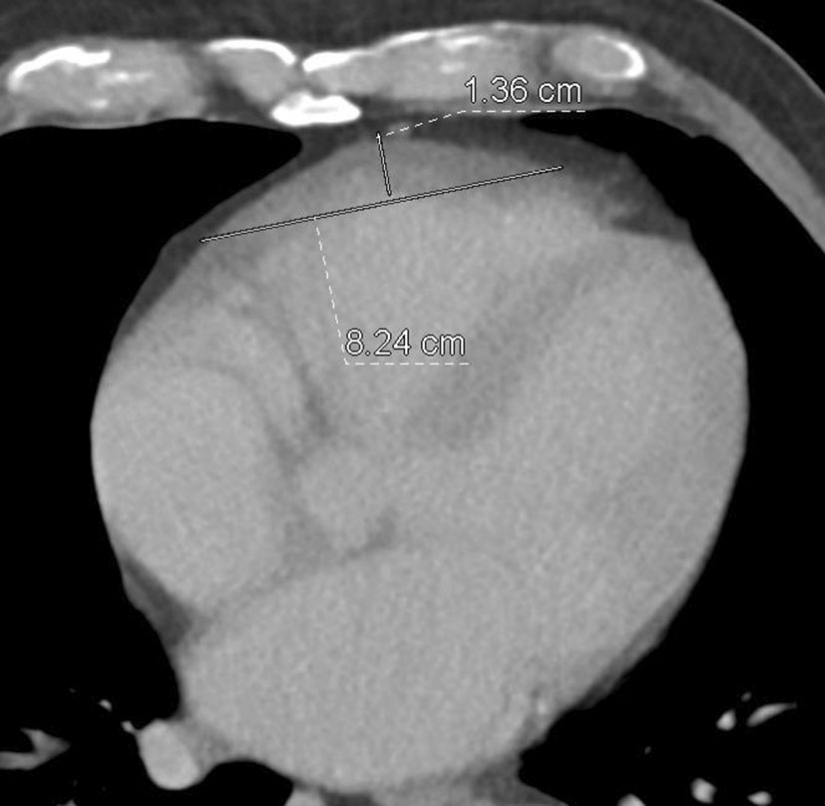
Cardiac CT shows a regredient wall thickening (8.2 × 1.3 cm) of the free wall of the right ventricle compatible with residual findings 10 months after therapy
